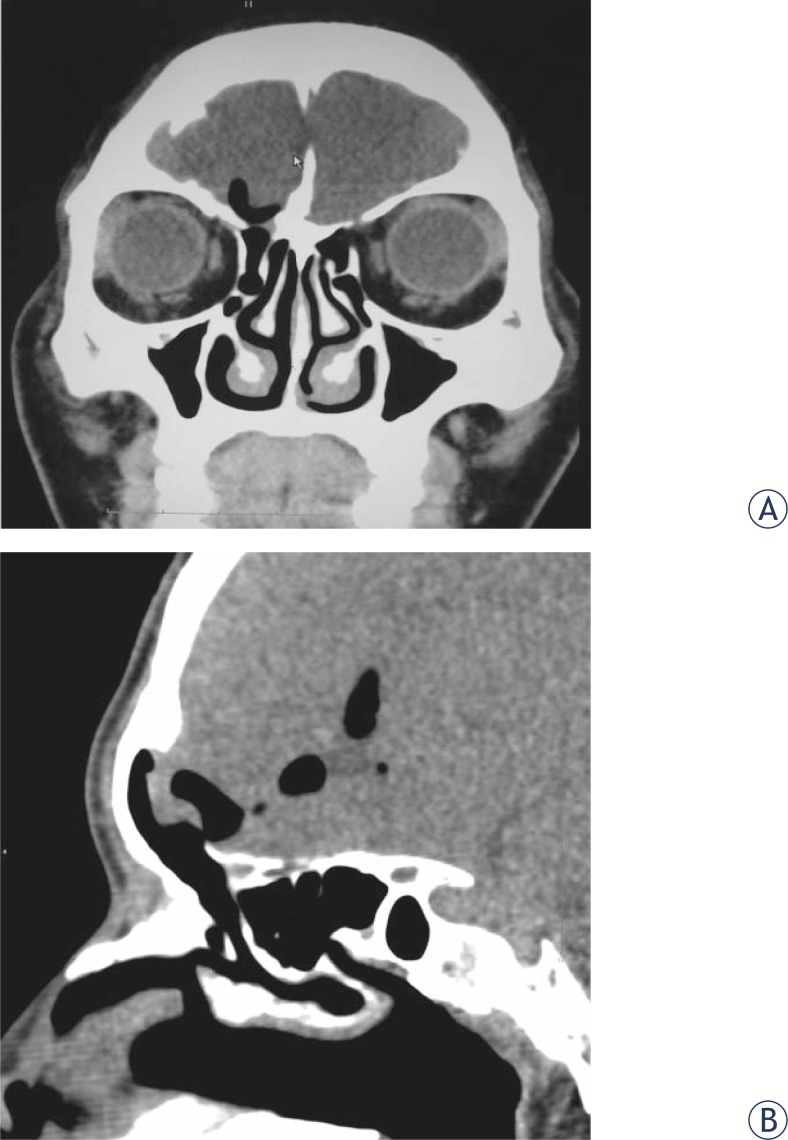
Cerebrospinal fluid rhinorrhea was present after brain trauma in a male patient of 37 year old. CT scan showed multiple basilar skull fracture. Thin layer scan of high-resolution CT showed that frontal sinus communicated with nasal cavity and cerebrospinal leak in frontal sinus was the diagnosis (A, coronal view; B, sagittal view). The surgical exploration confirmed that frontal sinus was impaired and communicated with intracalvarium and rhinorrhea disappeared after the surgical repair.
